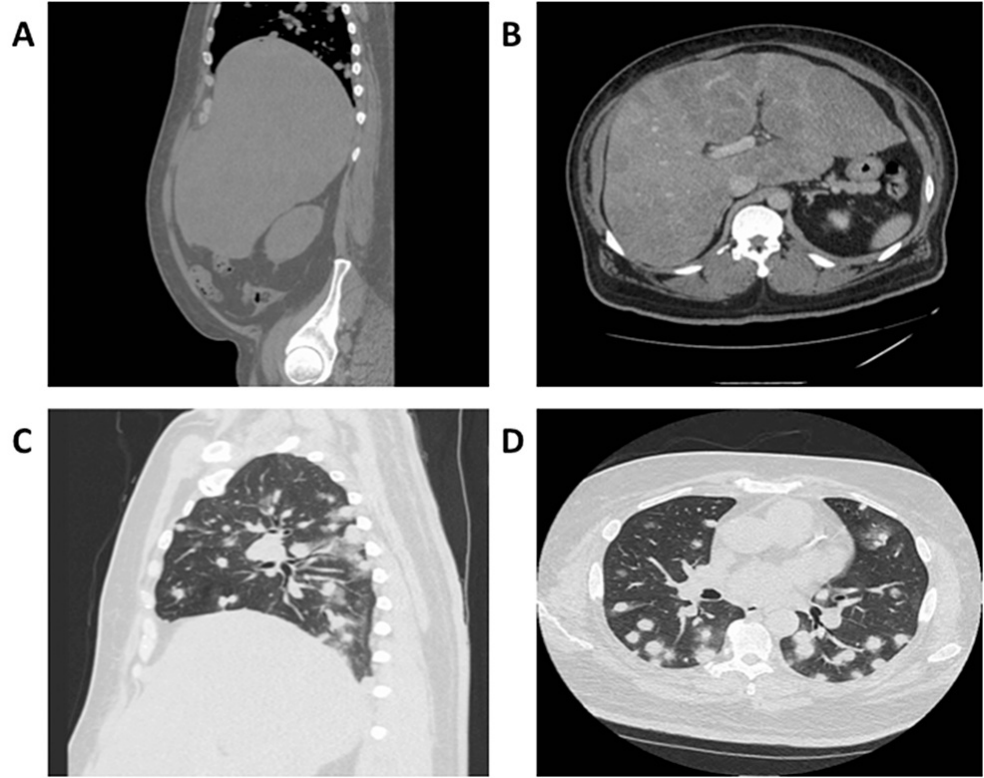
Abdomen/pelvis CT Scan: (A) sagittal and (B) transverse sections showing massive hepatomegaly with innumerable large ill-defined hypo-densities. Chest CT scan: (C) sagittal and (D) transverse sections showing innumerable pulmonary nodules. CT: computed tomography
